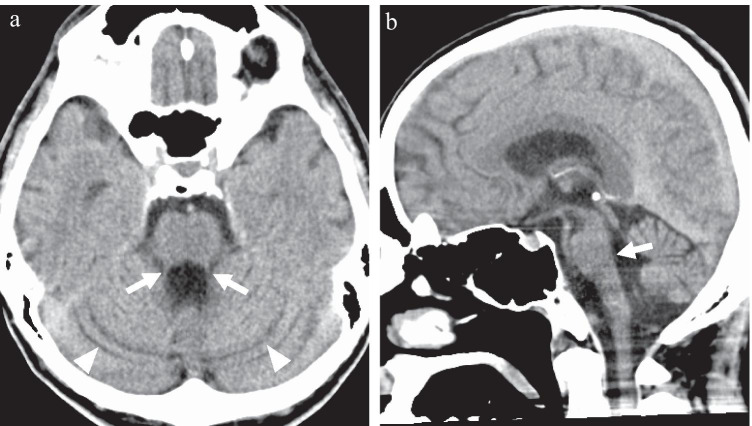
Brain computed tomography (CT). a Axial brain CT shows a mild enlargement of the bilateral cerebellar fissures (arrowheads), suggestive of cerebellar atrophy. The image also reveals bilateral superior cerebellar peduncle atrophy (arrow). b Sagittal brain CT demonstrates dilatation of the fourth ventricle, suggestive of tegmental atrophy of the pons (arrow)
